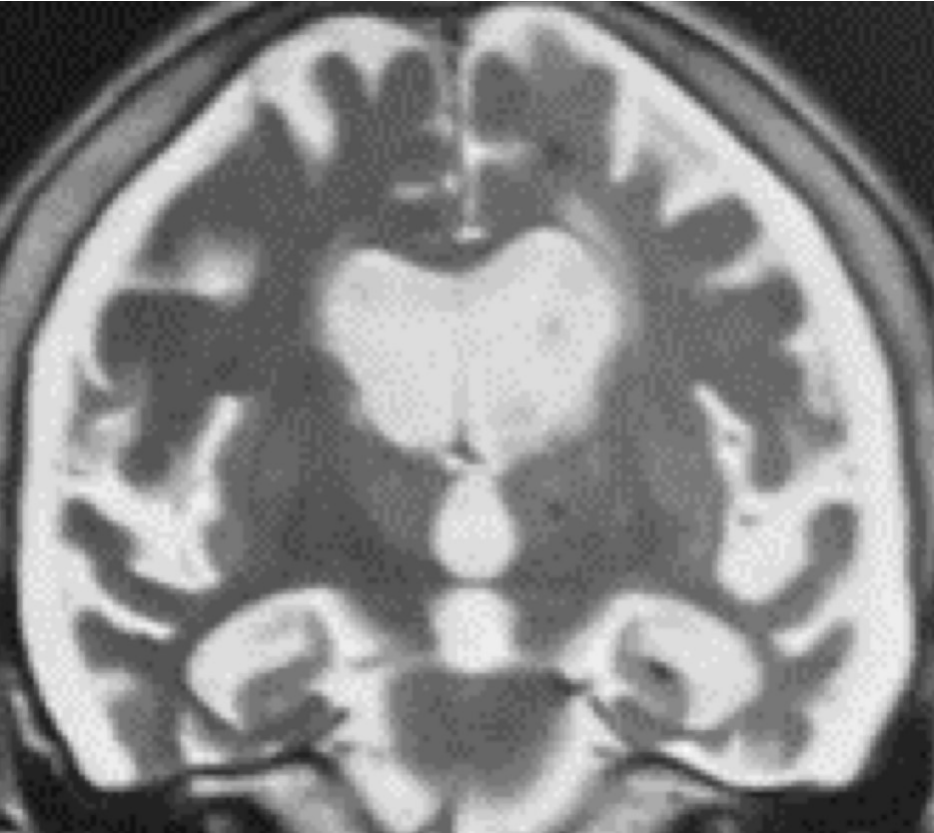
Coronal magnetic resonance imaging demonstrated diffuse cerebral atrophy slightly more prominent in the left hemisphere, the hippocampi showed a 3/4 Scheltens’ scale and extensive bilateral hemorrhagic subdural hematoma.
